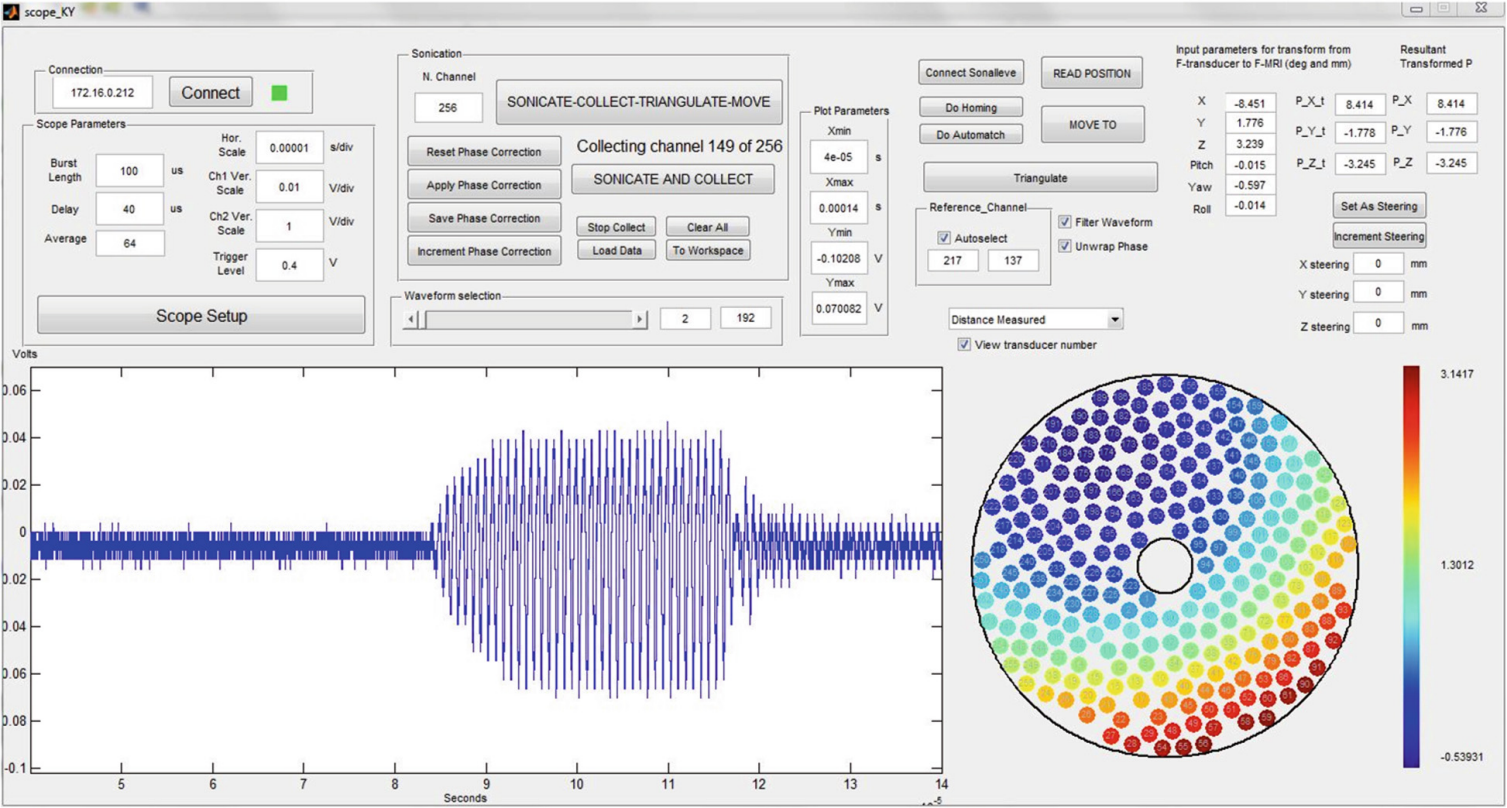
Graphical user interface used to perform ultrasound exposures and capture the acoustic hydrophone data. In this example, the acquisition 149 of 256 is being displayed.
